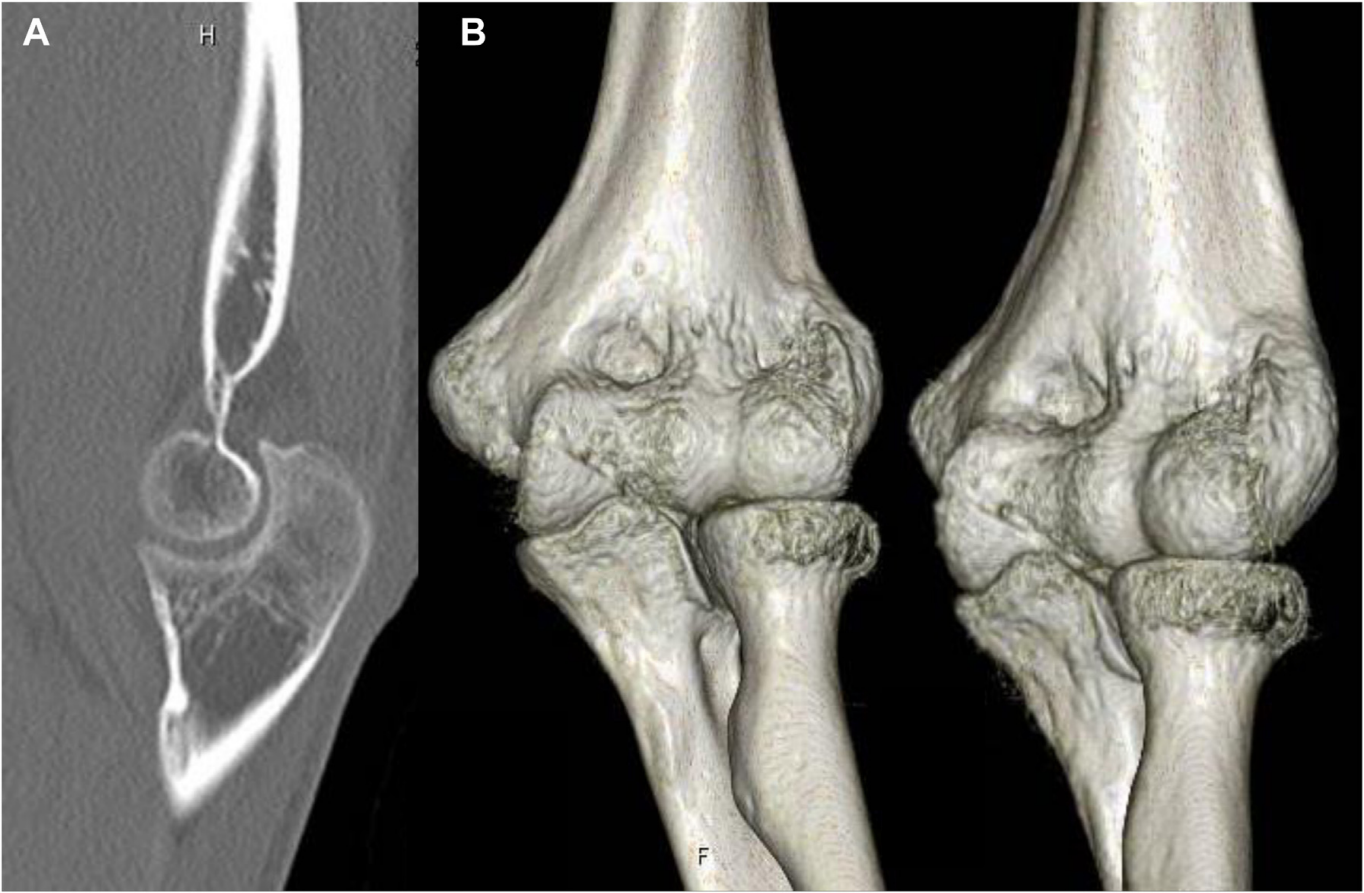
Computed tomography of the elbow joint 6 months after reduction. (A) Sagittal image and (B) 3-dimensional image. Bony fusion was confirmed with no dislocation.
